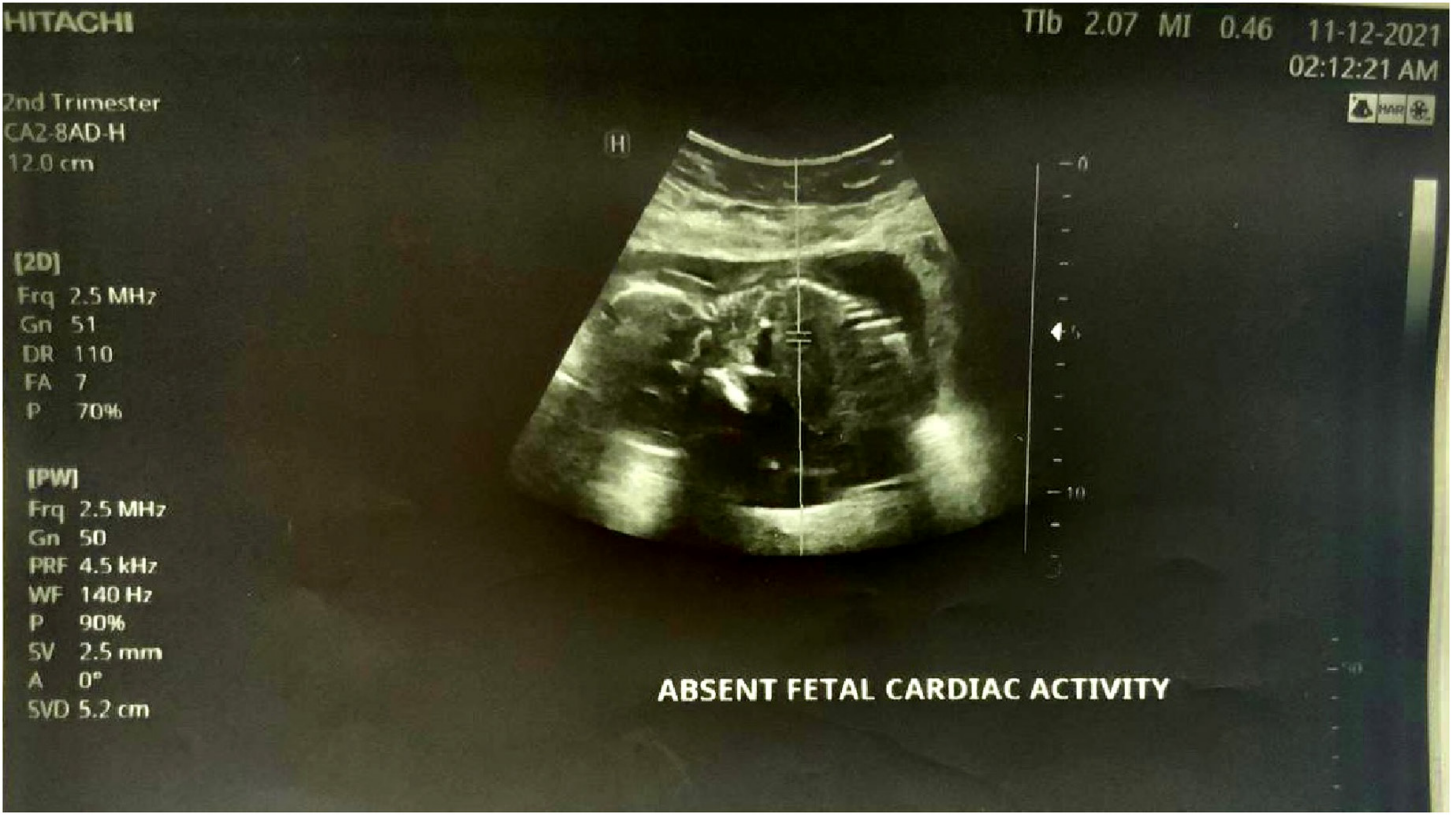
Ultrasound scan showing absent cardiac activity.
